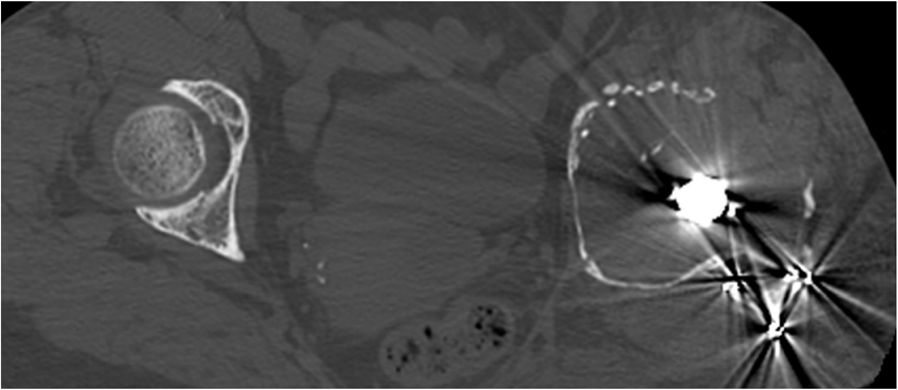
A plain computed tomography image of the bilateral hip in the axial section showing the fragmented anterior wall of the acetabulum and the extremely thin inner table
